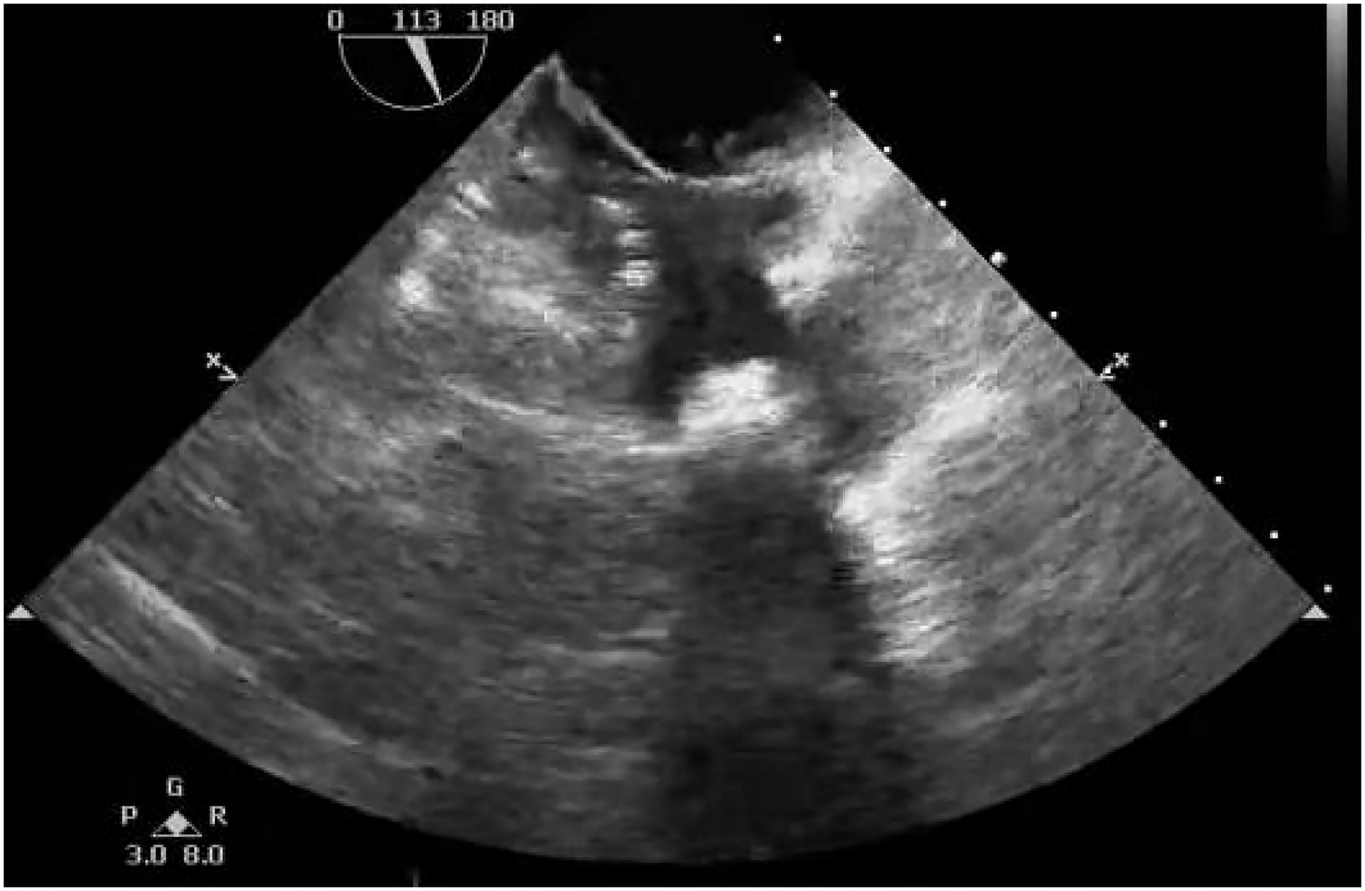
Postoperative transthoracic echocardiography showing that no residual shunt through the atrial septum was detected.
